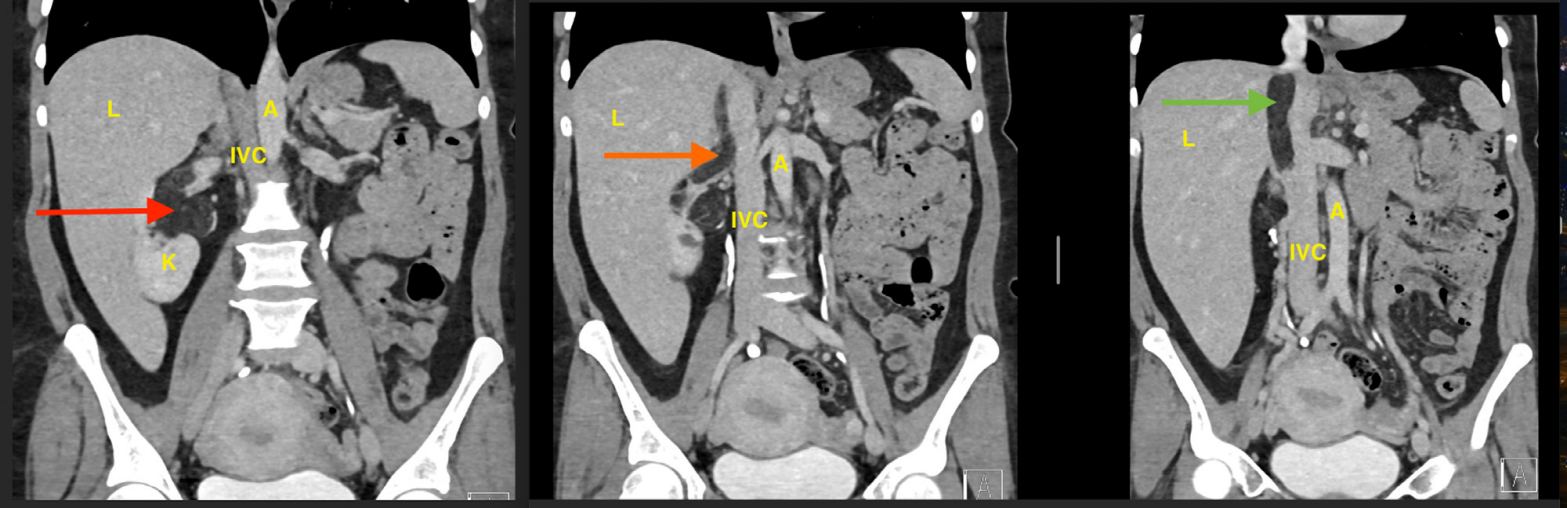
CT abdomen axial cut with contrast at 3 levels. • K: kidney. • L: liver. • A: abdominal aorta. • IVC: inferior vena cava. • Red solid-line arrows: the right renal mass abutting the pelvi-ureteric junction • Orange solid-line arrow: thrombus in the right renal vein • Green solid-line arrow: thrombus in the inferior vena cava.
